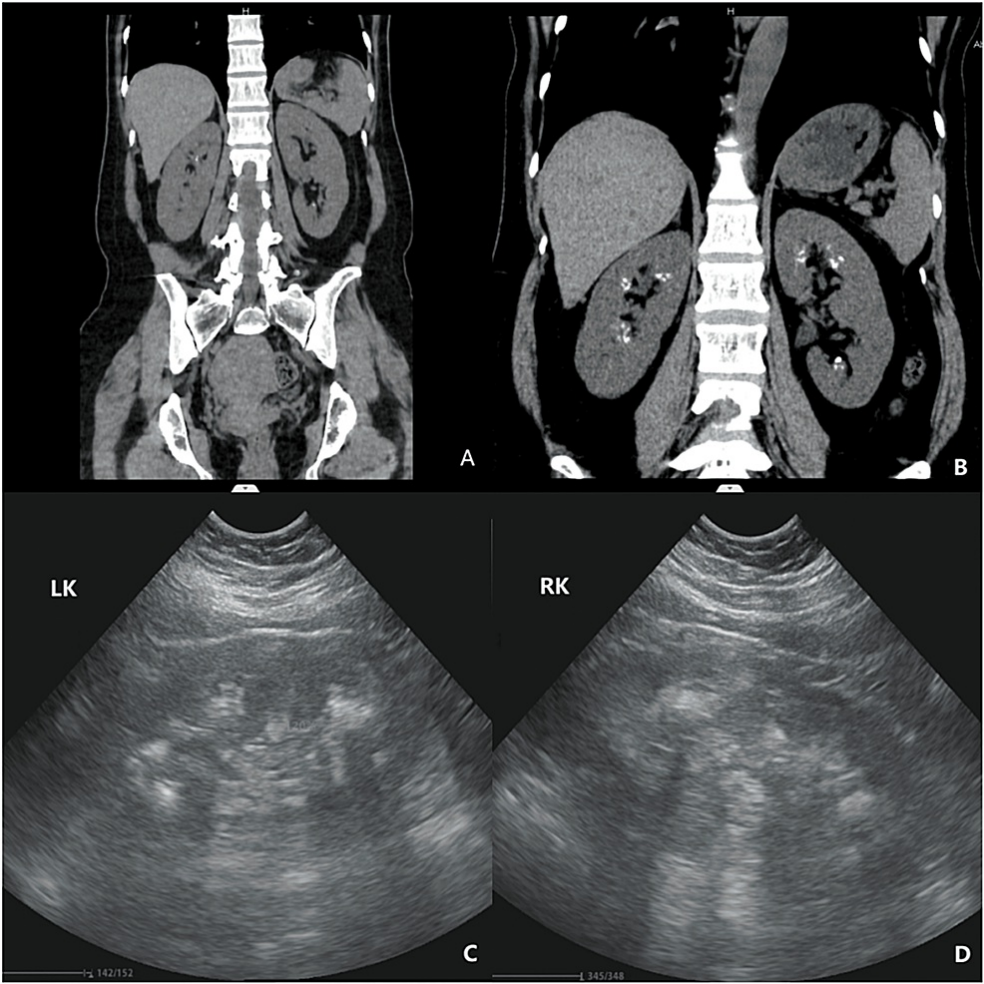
CT scan and color Doppler ultrasonography of both kidneys. (A) CT showing scattered stones in both kidneys. (B) CT showing high-density shadows in the renal medulla, with bouquet-like changes, indicating spongy kidneys. (C, D) Ultrasonography showing strong echoes, showing a fan-shaped arrangement, indicating spongy kidneys.
